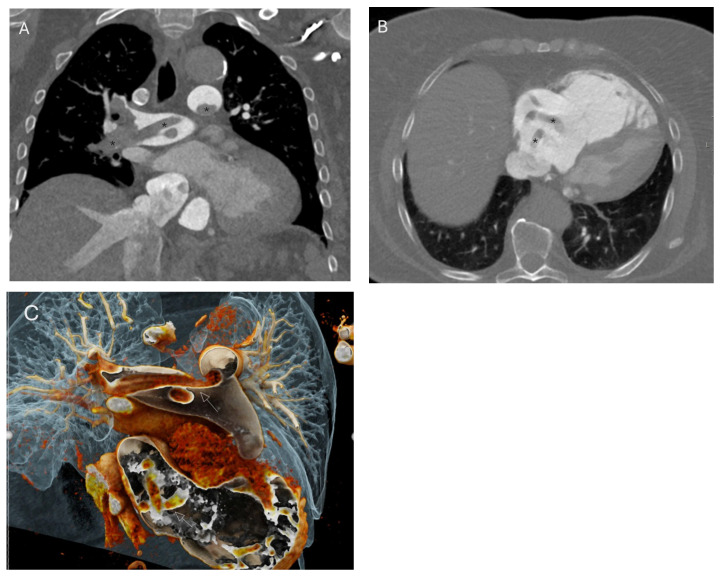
Computed tomography pulmonary angiography (CTPA) demonstrates contrast filling defects (annotated with stars, *) in the left and right pulmonary arteries, interlobar arteries, and the majority of segmental branches, consistent with a massive pulmonary embolism (A), as well as a serpiginous filling defect within the right atrium (B) consistent with clot-in-transit (CIT). Volume rendering reconstructions (C) show serpiginous thrombi in the main pulmonary arteries and right ventricle. CIT is a mobile thrombus temporarily lodged in the right heart, most commonly originating from deep pelvic veins, and can enter the pulmonary circulation at any moment, making it a medical emergency [1]. Therefore, early diagnosis is crucial, especially in haemodynamically unstable patients. The patient was a 70-year-old woman with a history of recent abdominal surgery who presented with acute dyspnoea and obstructive shock. Her blood pressure was 59/29 mmHg, pulse 90 beats per minute, and oxygen saturation (SpO_2_) 98%, with right heart enlargement, suggesting right heart strain.
